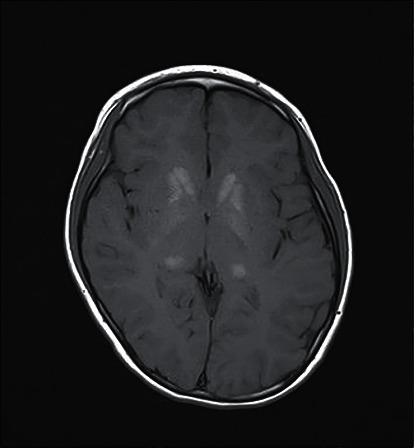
MRI brain showing T1 hyperintensities at caudate head, putamen, and thalamus caused by calcium.
